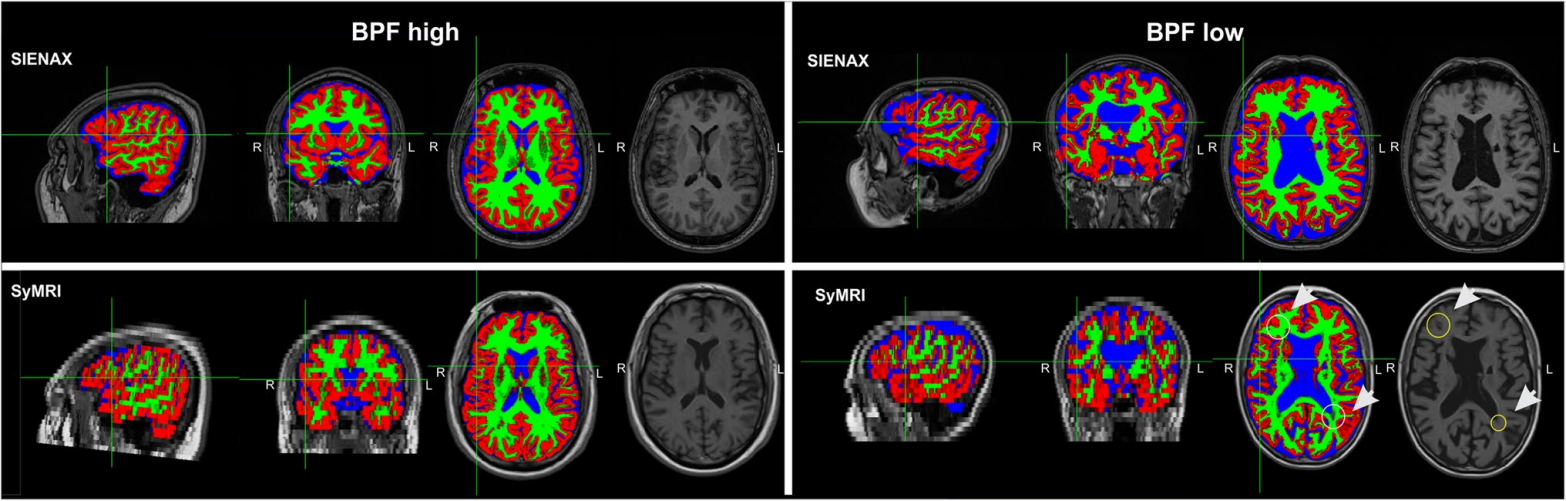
Comparison between brain segmentations with SIENAX and SyMRI in patients with low-grade brain atrophy (left column, termed “BPF high”) contrasted with marked brain atrophy (right column, termed “BPF low”); overlays shown on T1-weighted images represent the segmentation maps of white matter (green), grey matter (red), cerebrospinal fluid (blue); the right most columns show the corresponding T1-weighted images. Circles and white arrows in the right column point at examples of WM lesions in the synthetic T1-weighted images (without lesion filling) which are classified as GM in the SyMRI segmentation maps
